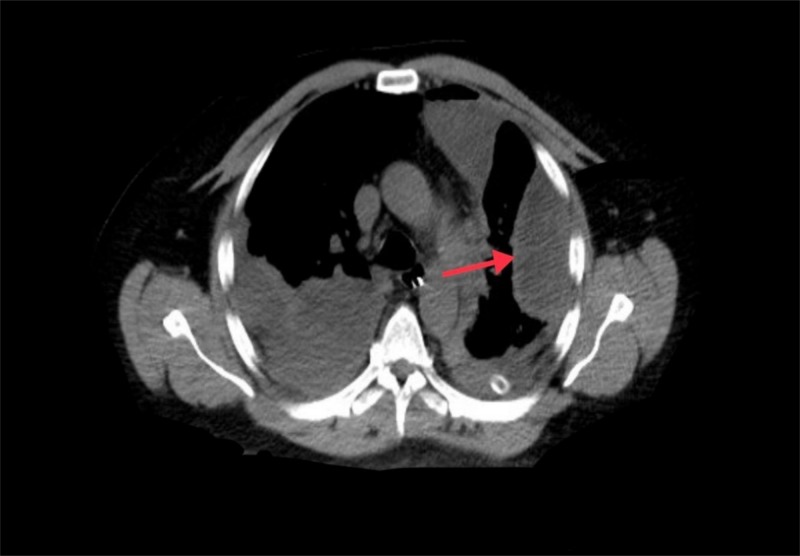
Chest CT scan Loculated abscess (red arrow)
